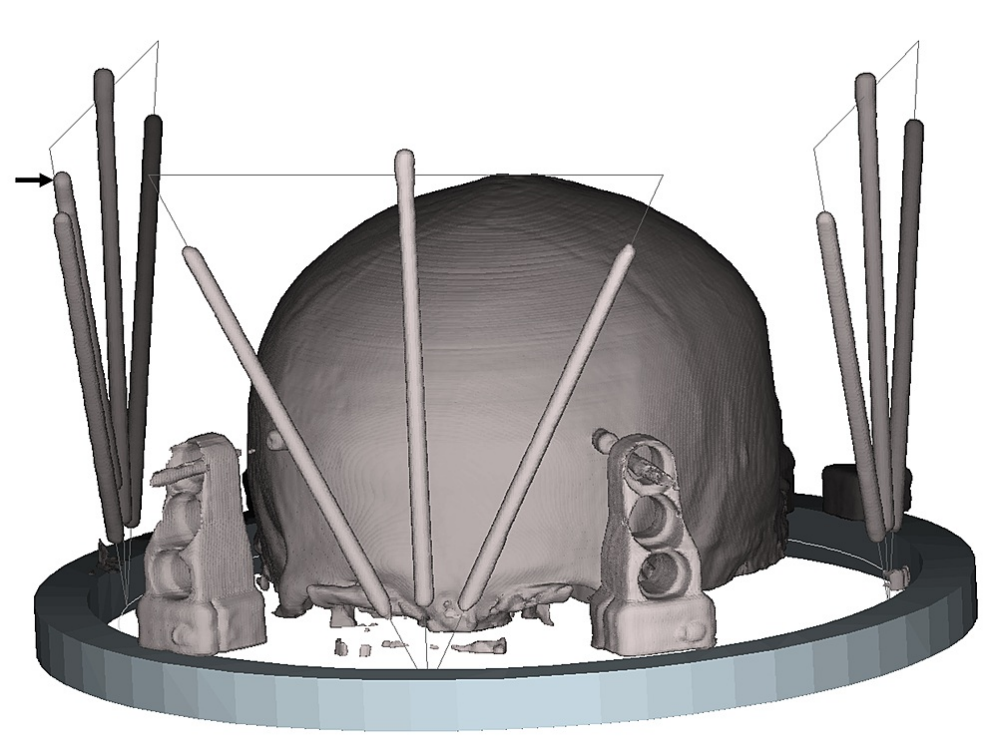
Three Sturm-Pastyr localizers attached to a Zamorano-Dujovny stereotactic frame A 3D reconstruction from a series of CT scan images shows three Sturm-Pastyr (SP) localizers attached to a Zamorano-Dujovny (ZD) stereotactic frame. The SP localizer attached to the right lateral aspect of the ZD frame includes an additional diagonal rod designated by the arrow. This rod produces in each CT scan image an ellipse that is not used to calculate \begin{document} \left( x,y,z \right) \end{document} coordinates but instead identifies the right lateral SP localizer in that CT scan image.
